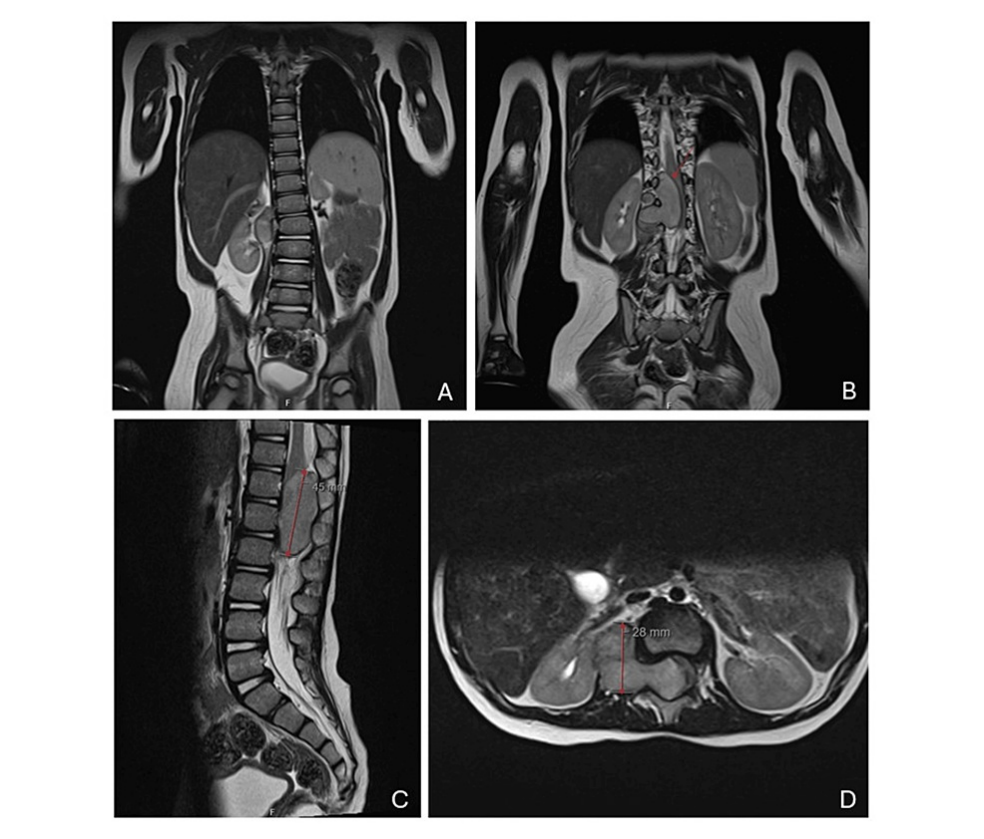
(A) Coronal view showing a slight dorsolumbar scoliosis. (B) Coronal view showing an expansive formation in the dorsolumbar and right lateral paravertebral region. (C) Sagittal view. (D) Axial view of the neuraxial MRI. A large expansive formation in the dorsolumbar and upper lumbar region, occupying both the endocanal and extramedullary compartment and the right lateral paravertebral region, continuing via the intervertebral foramen to the right, especially at the L1-L2 level.
